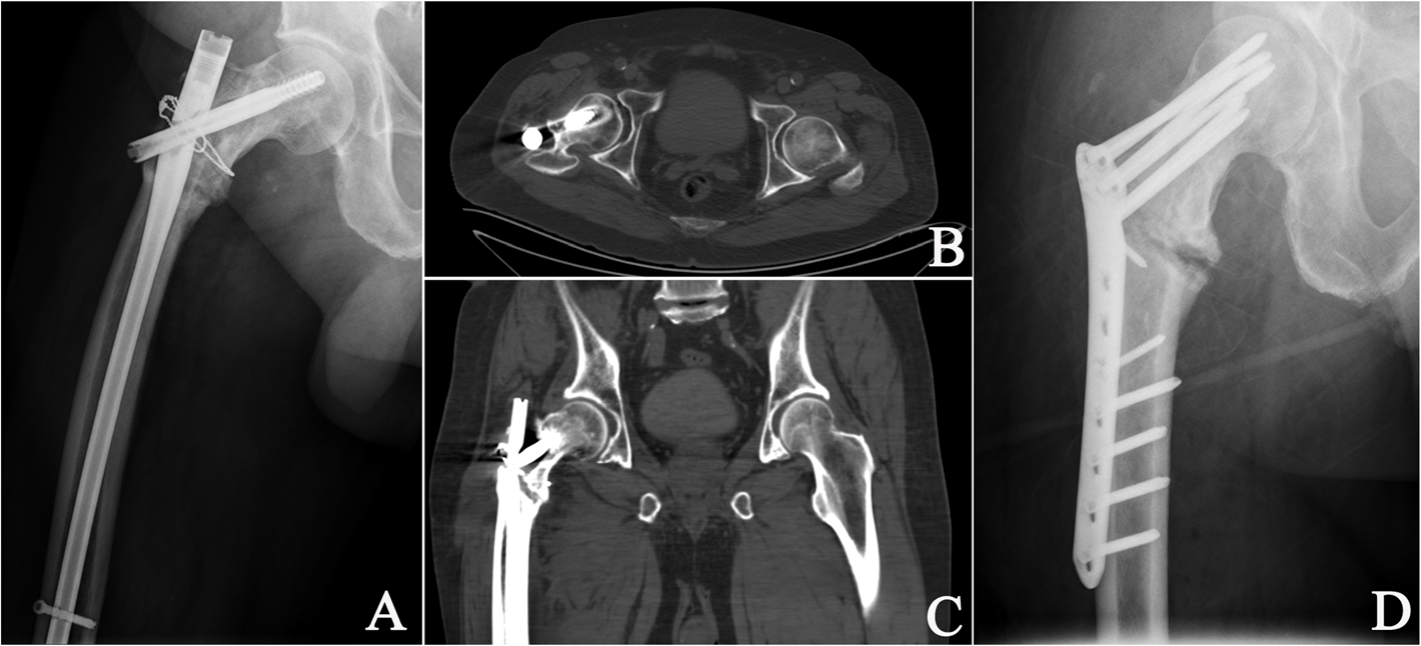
Failed nailing of an intertrochanteric fracture in an active 78-year-old man. a The intertrochanteric fracture had been stabilized by an antegrade long γ nail and circumferential cerclage 4 years ago. The patient first experienced significant hip pain and restricted hip motion 1 month ago without trauma history. Radiography showed nonunion of the intertrochanteric fracture and breakage of the γ nail. b Transverse and c coronal sections of CT scans showed osteolysis of the great trochanter as well as coxa vara with obvious fracture gaps. d Prior implants were removed. The malalignment was corrected, causing a larger gap in the calcar. Sufficient bone grafting was used to fill the gap, and the fracture was stabilized by a reverse LISS for the distal femur
